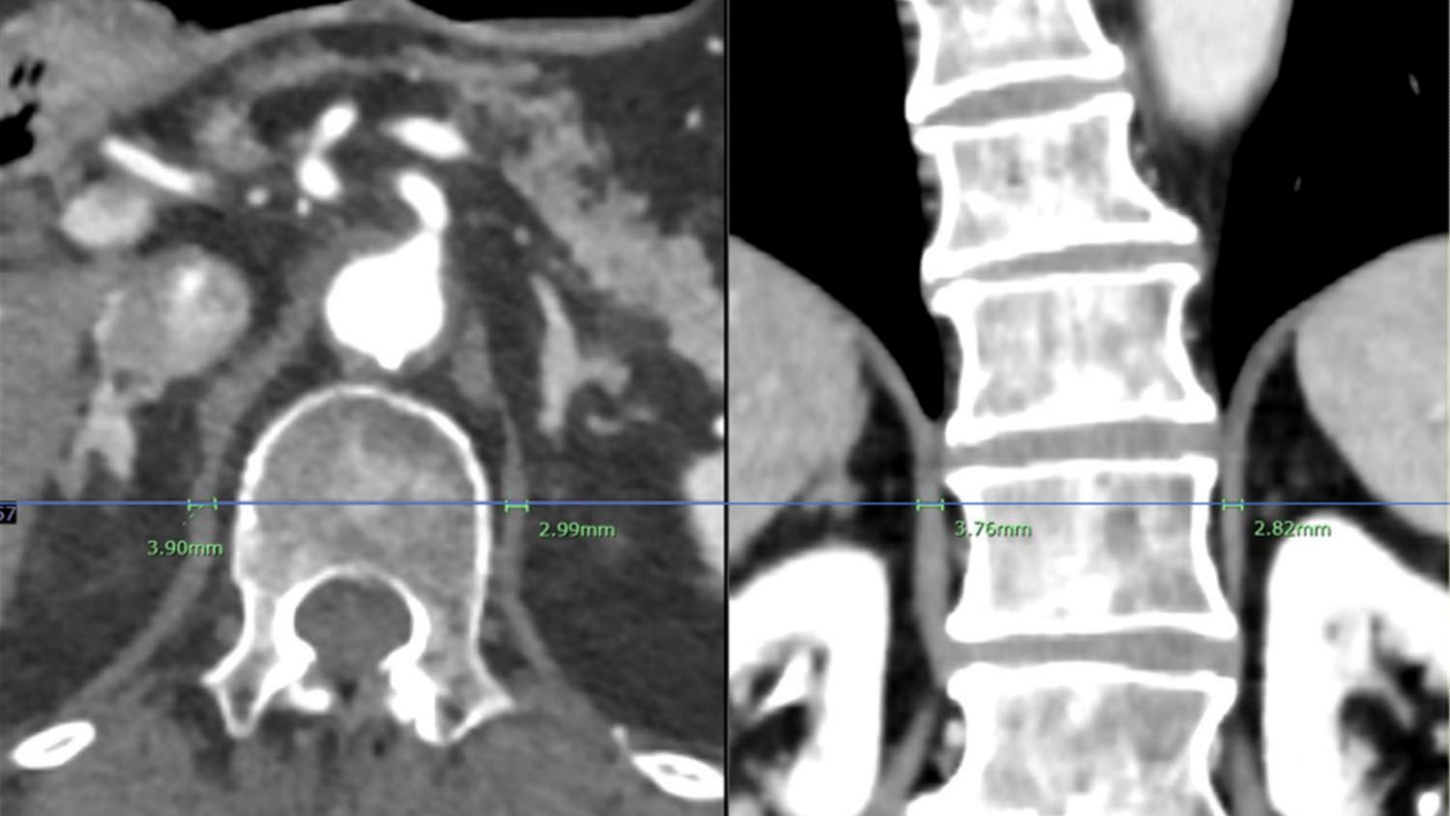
Measurement of both hemidiaphragm thicknesses at the middle vertebral body at the level of the celiac artery origin on axial and coronal CT
